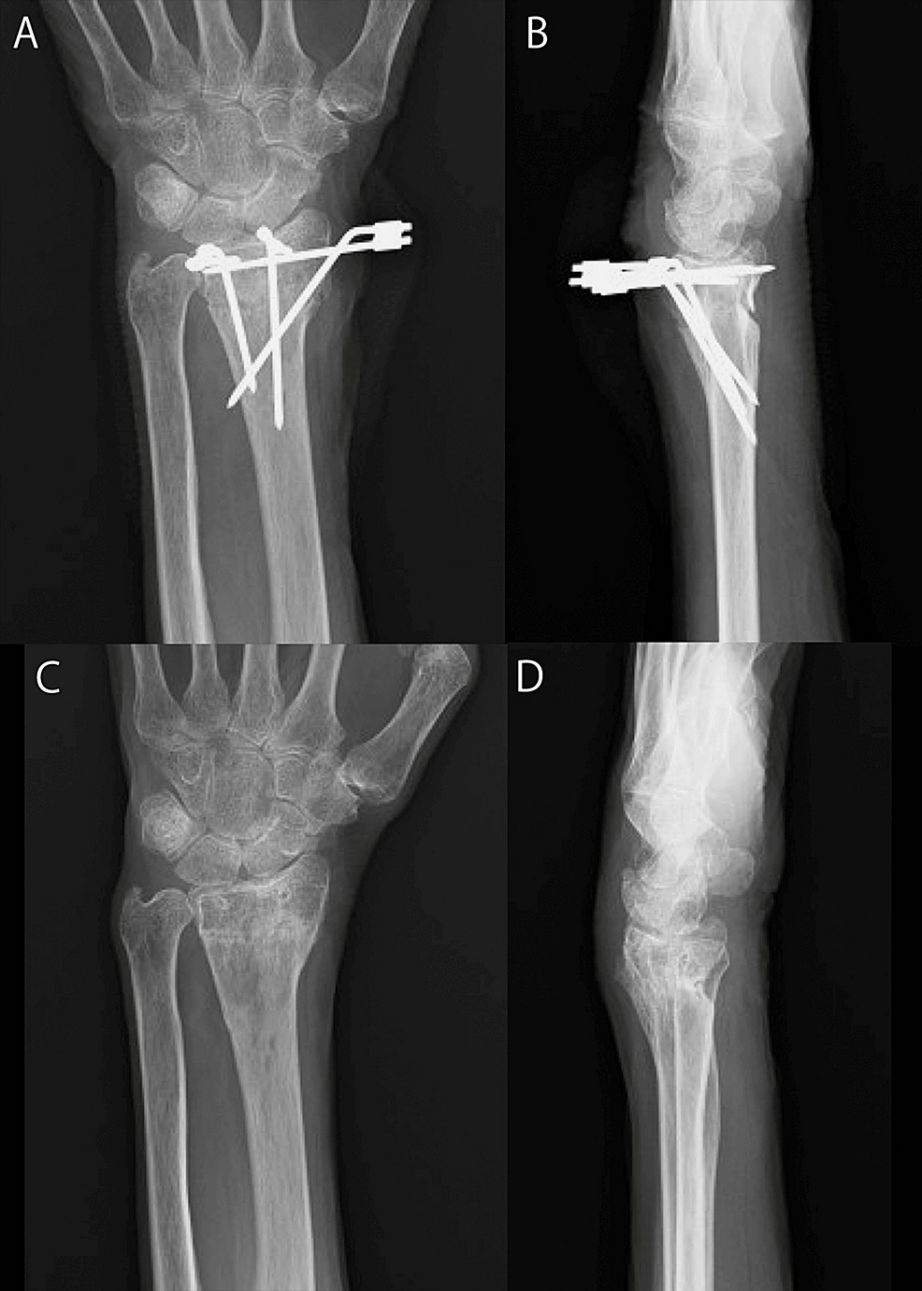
Plain radiography after osteosynthesis with a locked wire fixator. Frontal view (A) and lateral view (B) after osteosynthesis. Frontal view (C) and lateral view (D) 12 months after osteosynthesis.
